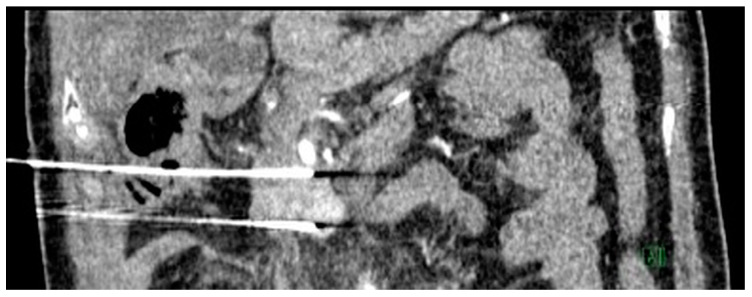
Intra-operative coronal computed tomography (CT) image demonstrating two irreversible electroporation (IRE) probes inserted in the cranio-caudal orientation into the tumor bed. Percutaneous IRE procedural details: Two IRE probes were inserted in the cranio-caudal orientation into the tumor bed under CT guidance, ensuring the needle is 2 cm apart and parallel—unsheathed 1.5 cm. Two cycles of 90 pulses at 2500 V performed with 12 A rise in current (initial 10 test pulses performed). This was followed by withdrawal of the needles proximally by 1.5 cm with another two cycles of 90 pulses. Post-ablation scan showed no active bleed and air locules seen around the surgical bed secondary to hydrolysis. Needles were then removed.
